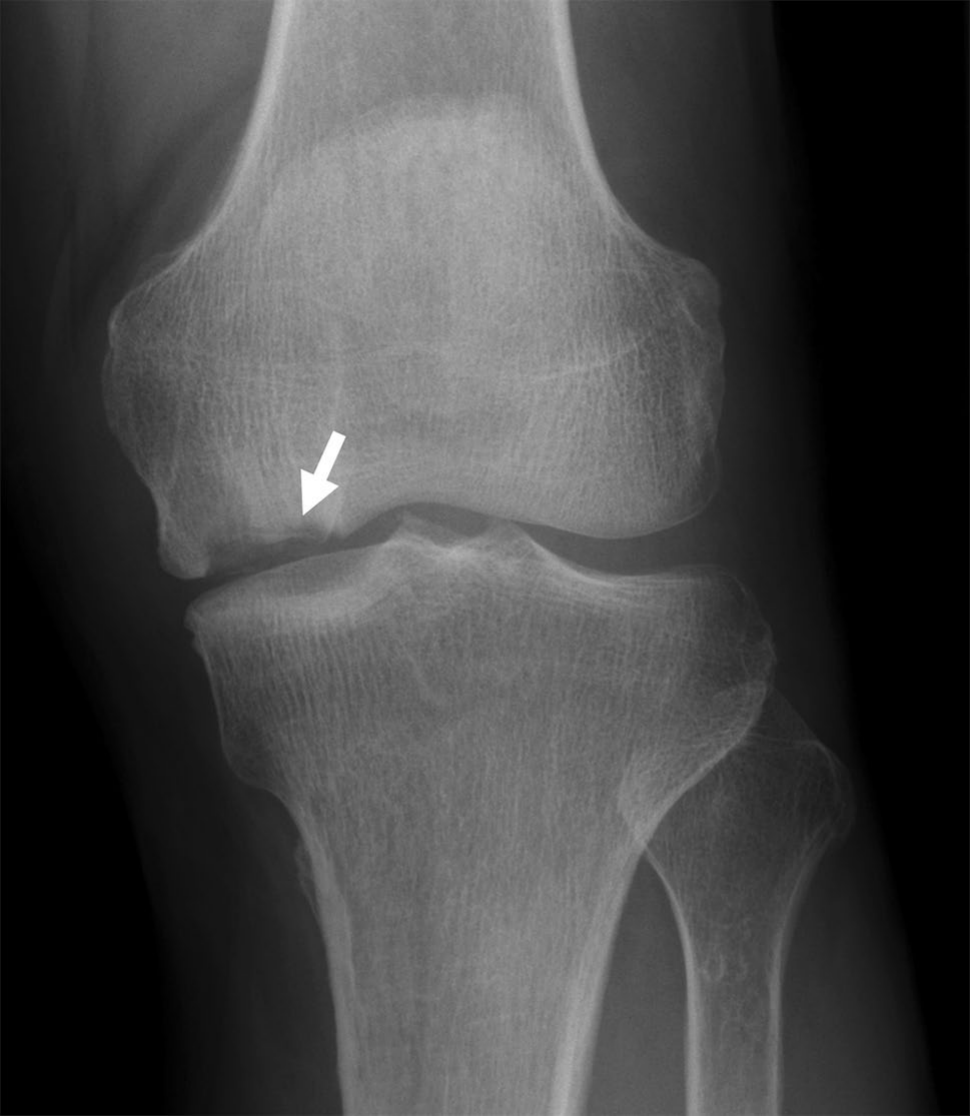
Plain radiograph of SIFK in a 75-year-old female patient. Saucerized defect (arrow) of the epiphysis can be observed in the medial femoral condyle of the left knee
